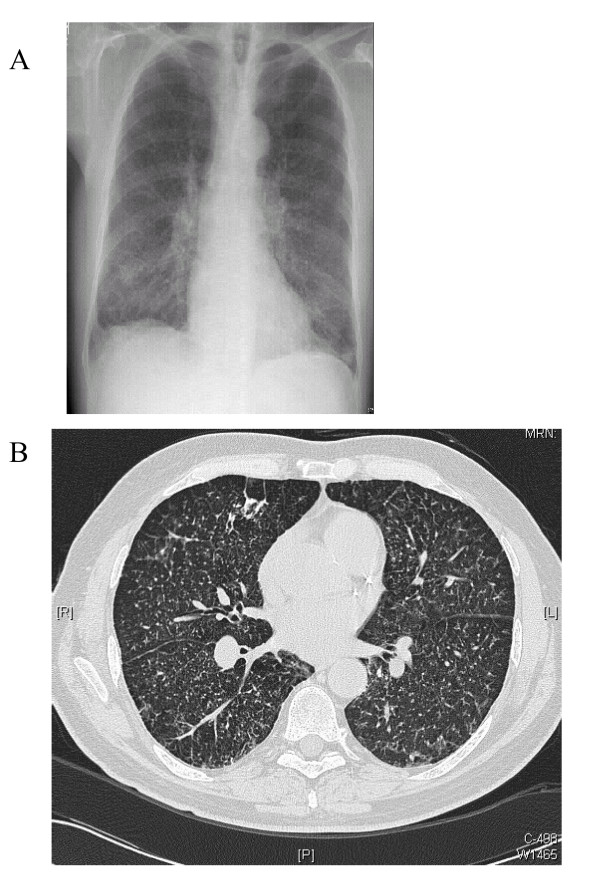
Chest radiograph and computed tomography. (a) Chest radiograph demonstrating diffuse generalised reticular nodular shadowing. (b) Chest computed tomography scan showing bilateral tiny nodular opacities throughout both lung fields predominantly in the mid and upper zones.
